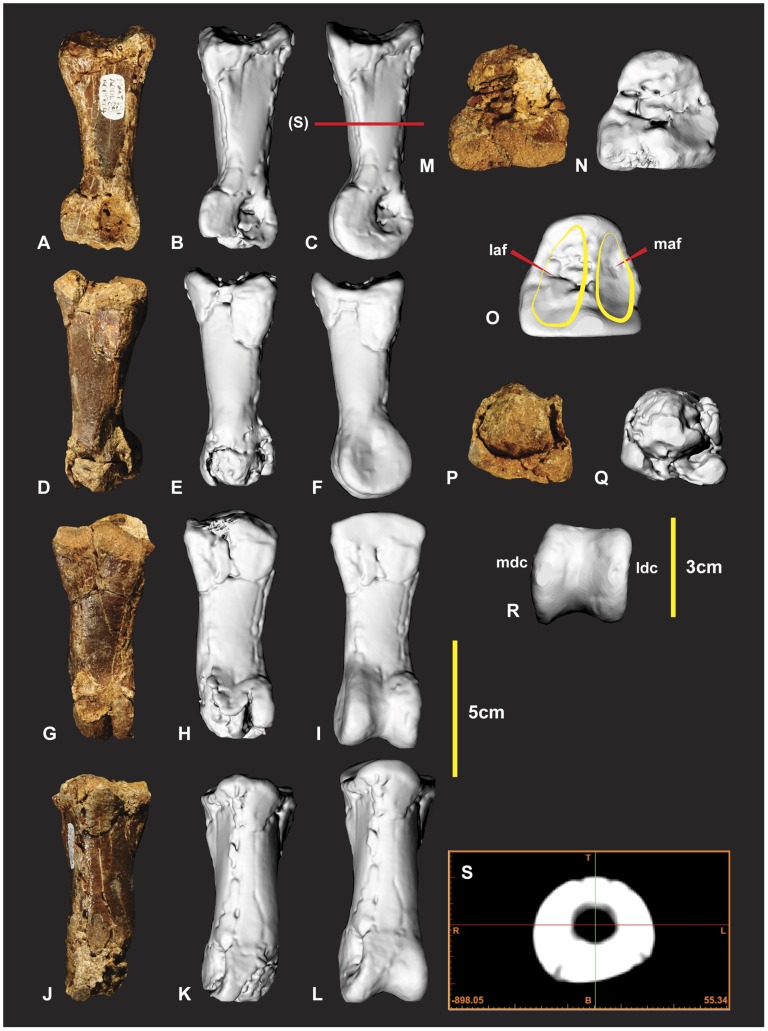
Left manual phalanx II-2. Left manual phalanx II-2 in medial (A, B & C), lateral (D, E & F), ventral (G, H & I), dorsal (J, K & L), proximal (M, N & O), distal (P, Q & R), mid shaft CT image (S). Computer generated renders with modified clay surfaces to represent missing elements are displayed in (C, F, I, L, O & R) views. *Abbreviations:* laf, lateral articular facet; ldc, lateral distal condyle; maf, medial articular facet; mdc, medial distal condyle.
